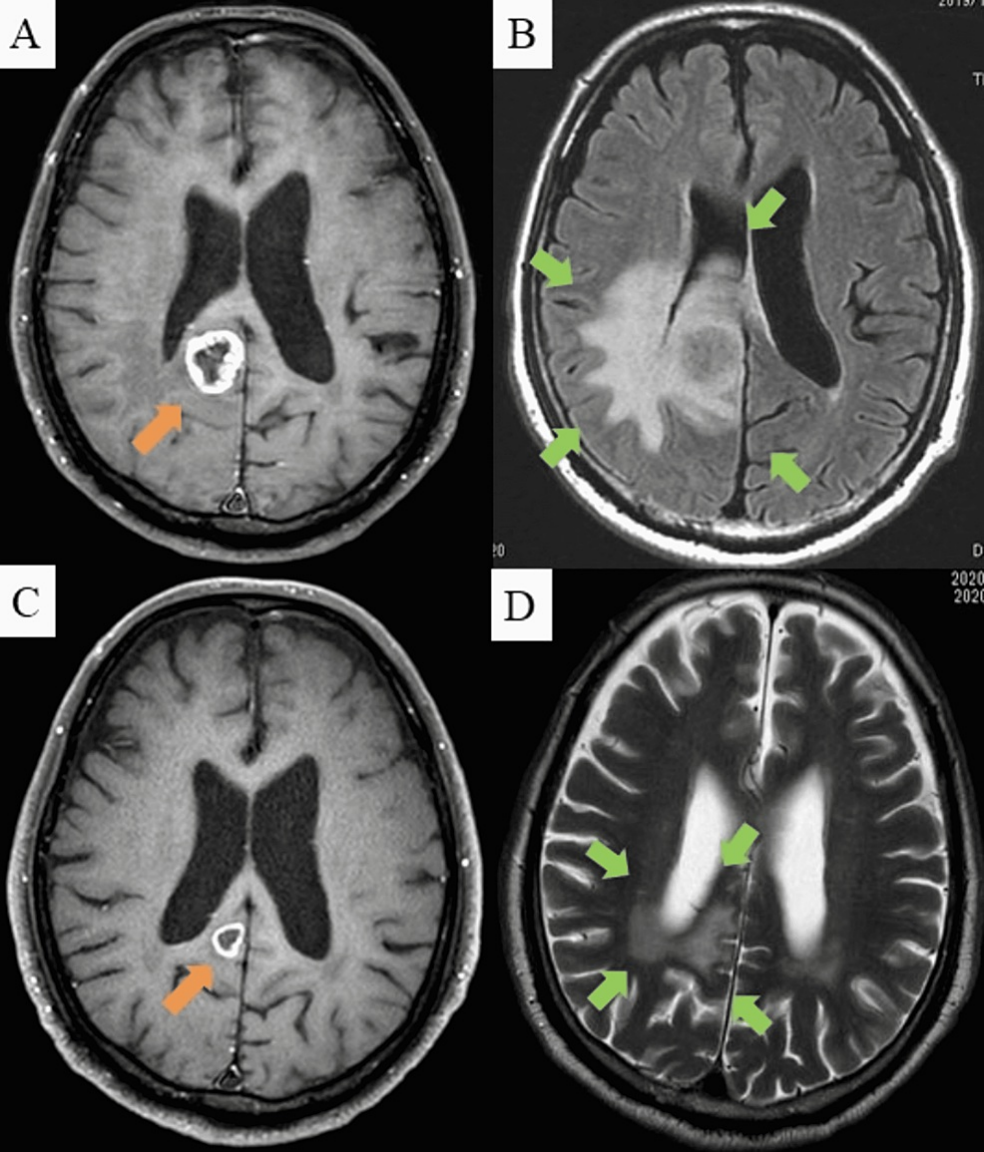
Magnetic resonance image of brain. (A) Contrast-enhanced magnetic resonance image revealing a 2-cm mass with ring enhancement in the right parietal lobe in the post-contrast T1-weighted condition (orange arrow). (B) Extensive vasogenic edema (green arrows) was confirmed by fluid-attenuated inversion recovery. (C) Reduced tumor with ring enhancement (orange arrow) and (D) reduced vasogenic edema (green arrows) after radiotherapy.
